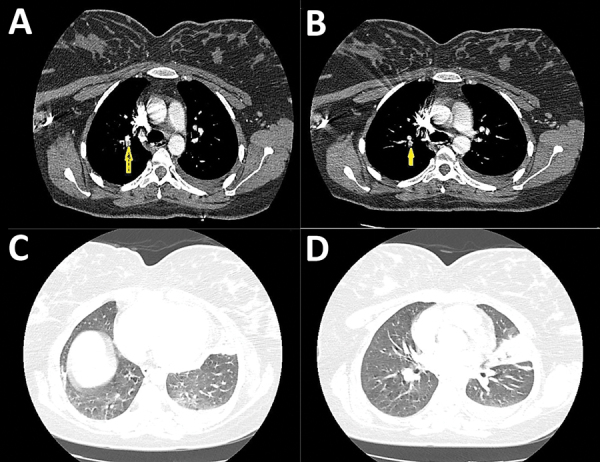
Thoracic computed tomography angiography of a 36-year-old postpartum woman with coronavirus disease and acute pulmonary embolism, Iran. A, B), Thoracic computed tomography angiography showing filling defect in the right side inter-lobar artery (arrow) and posterior basal segment and left-sided pleural effusion (arrow). C, D) Consolidation and ground-glass opacities affecting the left ligula and posterior recess.
